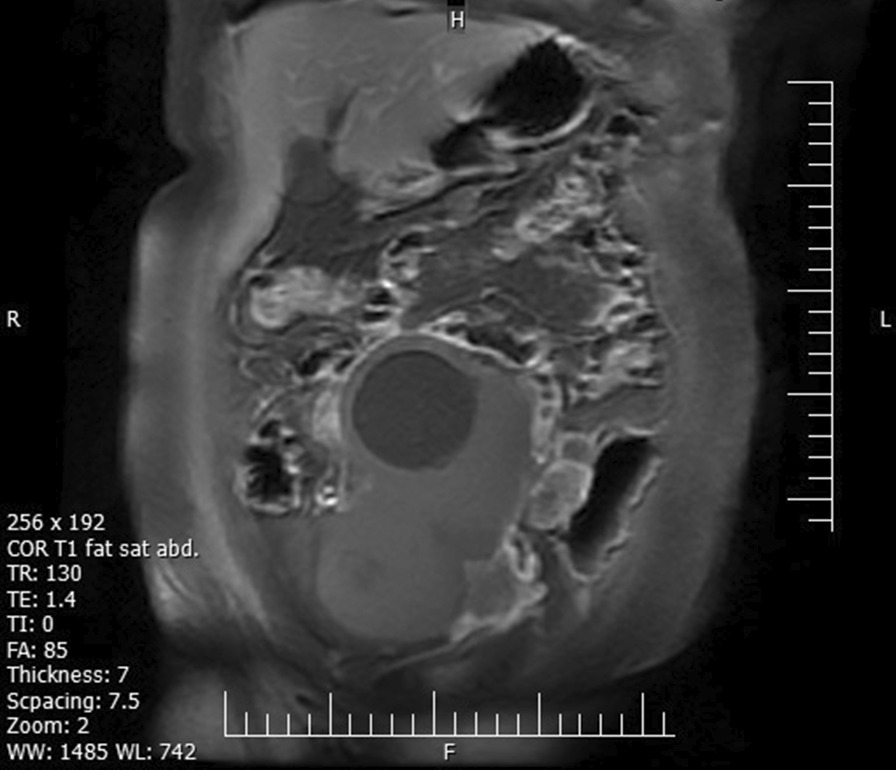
Coronal T1-weighted magnetic resonance imaging image with fat saturation showing an intra-abdominal large solid-cystic mass, with fat foci and omental thickening in the vicinity of the tumor
